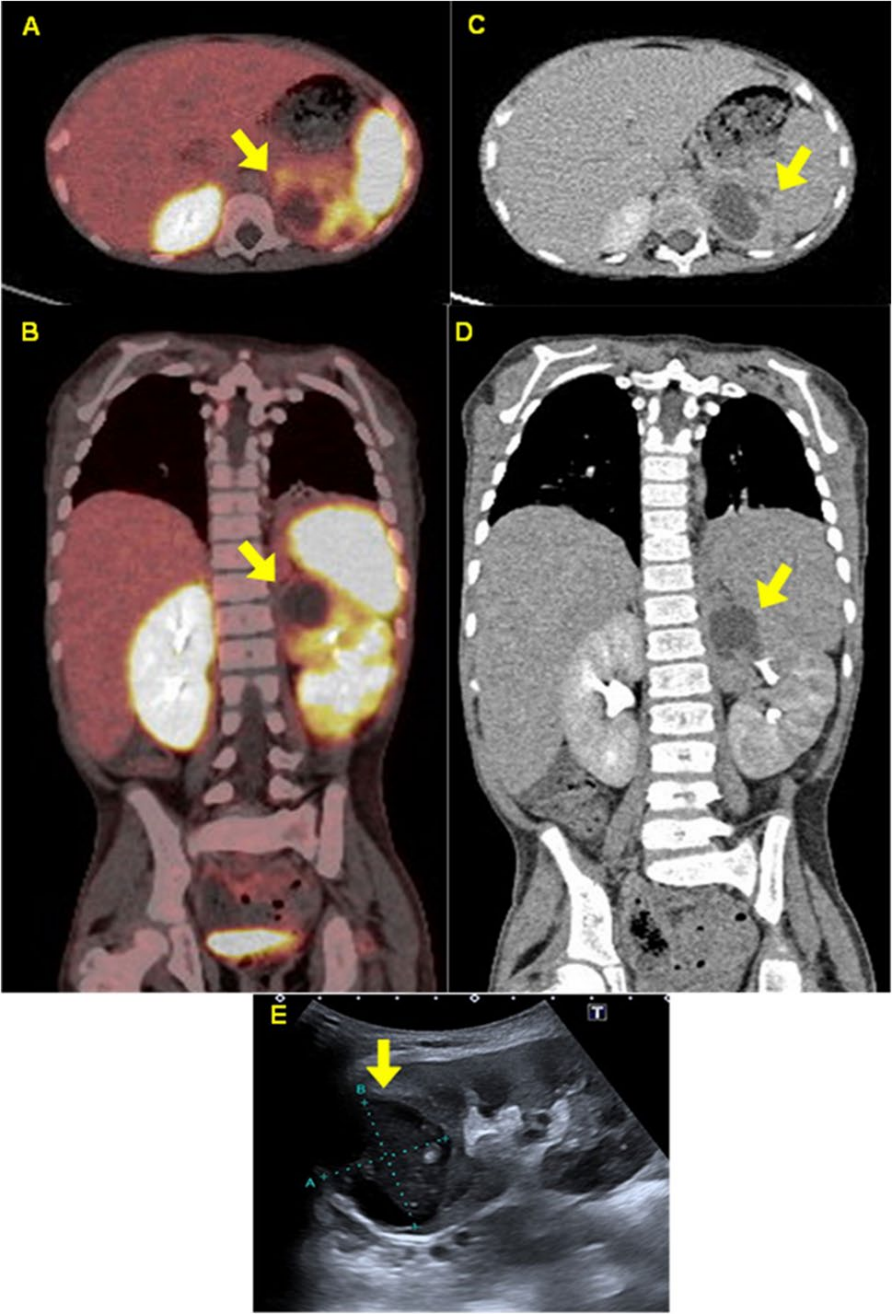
This series of images demonstrates the XGP (arrows) on axial (A) and coronal (B) views of the Ga-68-DOTATE positron emission tomography, axial (C) and coronal (D) views of the computerised tomography, and an ultrasound image (E). Note the lower uptake of tracer in the affected kidney, pronounced hepatomegaly, and the fusion of some of the vertebrae
